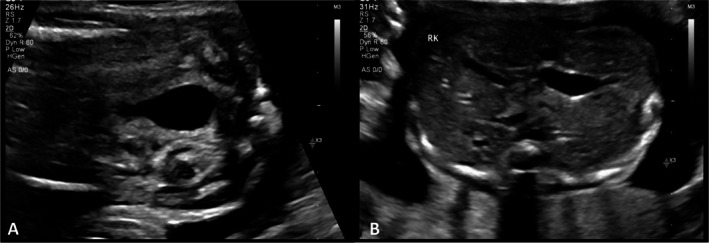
Prenatal ultrasound findings in Case 1. (A) Enlarged fetal bladder with thickened wall and “keyhole sign” at 20 weeks' gestation. (B) Bilateral hydronephrosis with normal renal echogenicity and no cortical cysts.
